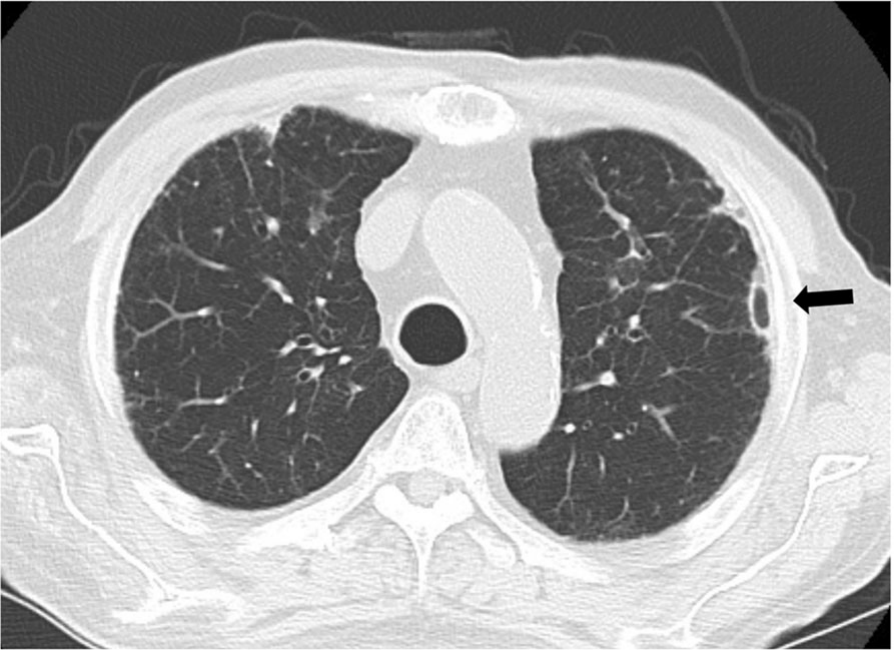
Chest computed tomography before pneumothorax showing a subpleural nodule with cavitation (arrow)
